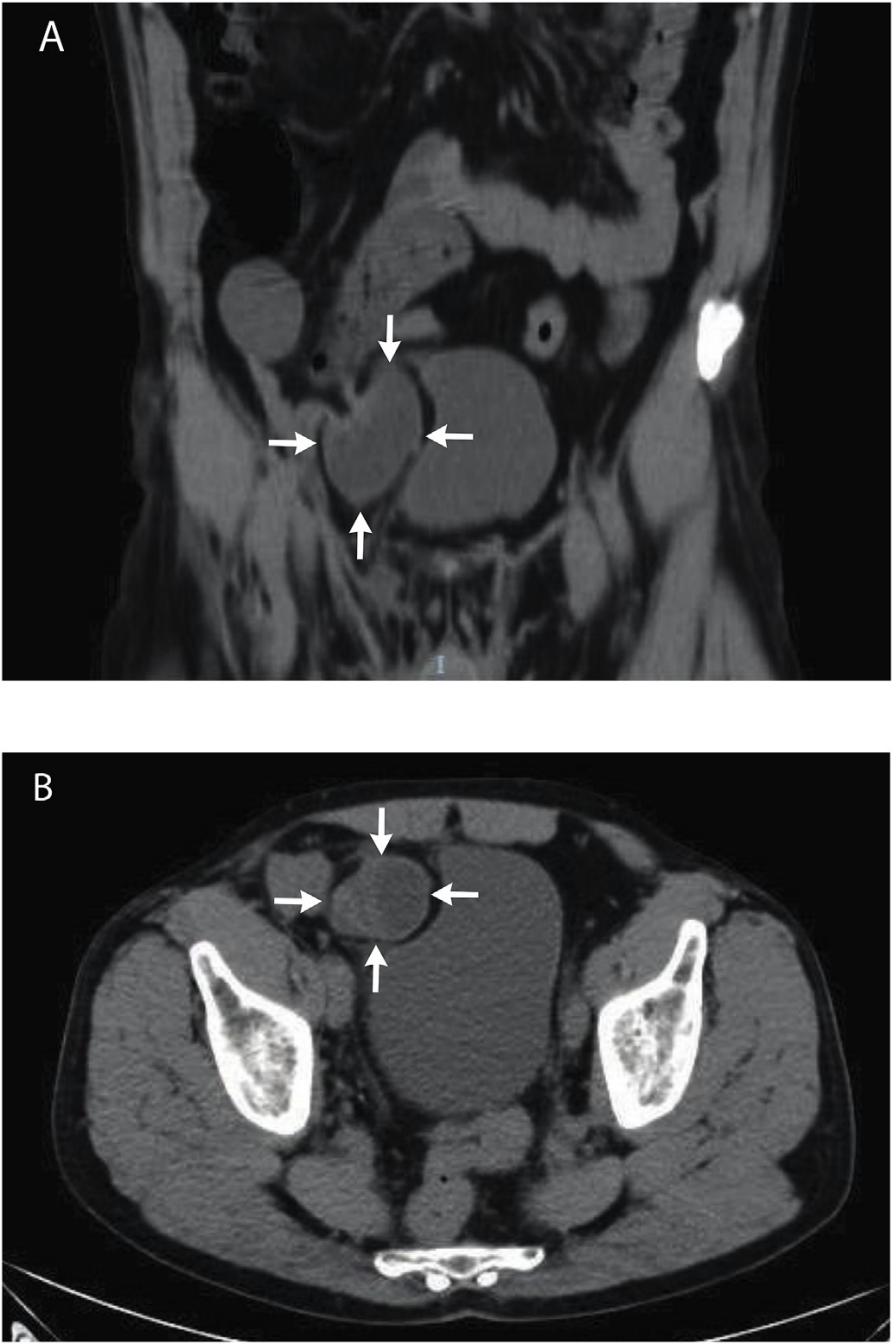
Abdominal CT scan showing a saclike mass (arrows) of bowel loops within a hernia sac below the transitional zone, indicating an internal hernia. Coronal (A) and sagittal (B) section.
